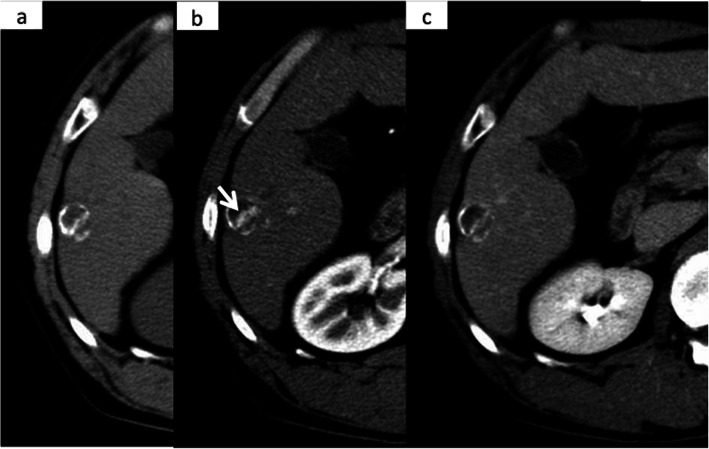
Dynamic CT. a A calcified tumor was seen in segment 6 of the liver in the plain CT. b CT in the arterial phase showing partial early enhancement of the tumor (indicated by white arrows). c CT in the delayed phase showing a washed-out pattern in the tumor
